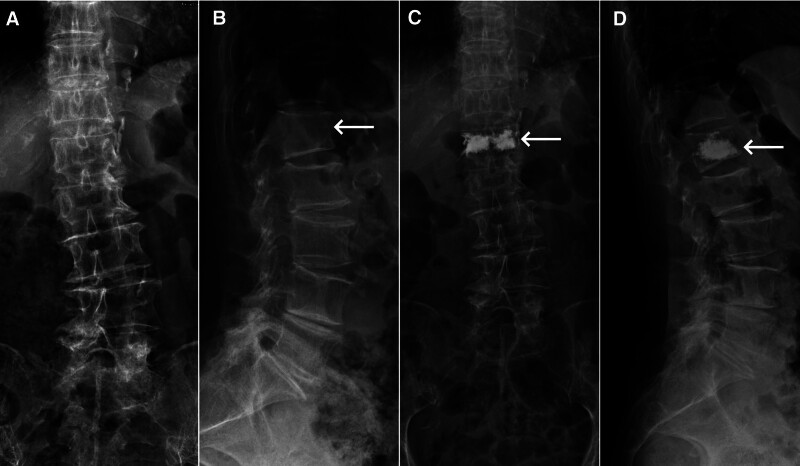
(A) Preoperative anteroposterior radiography showed lumbar degeneration with mild lateral curvature. (B) Preoperative lateral radiography revealed wedge-shaped deformation of the L1 vertebral body (arrow), showing anterior height loss without posterior rupture; no fracture fragments penetrating the spinal canal were observed. (C, D) Postoperative anteroposterior (C) and lateral (D) radiographies exhibited a mass-like high-density shadow (arrow) within the L1 vertebral body, with no free high-density shadows observed around the vertebral body.
